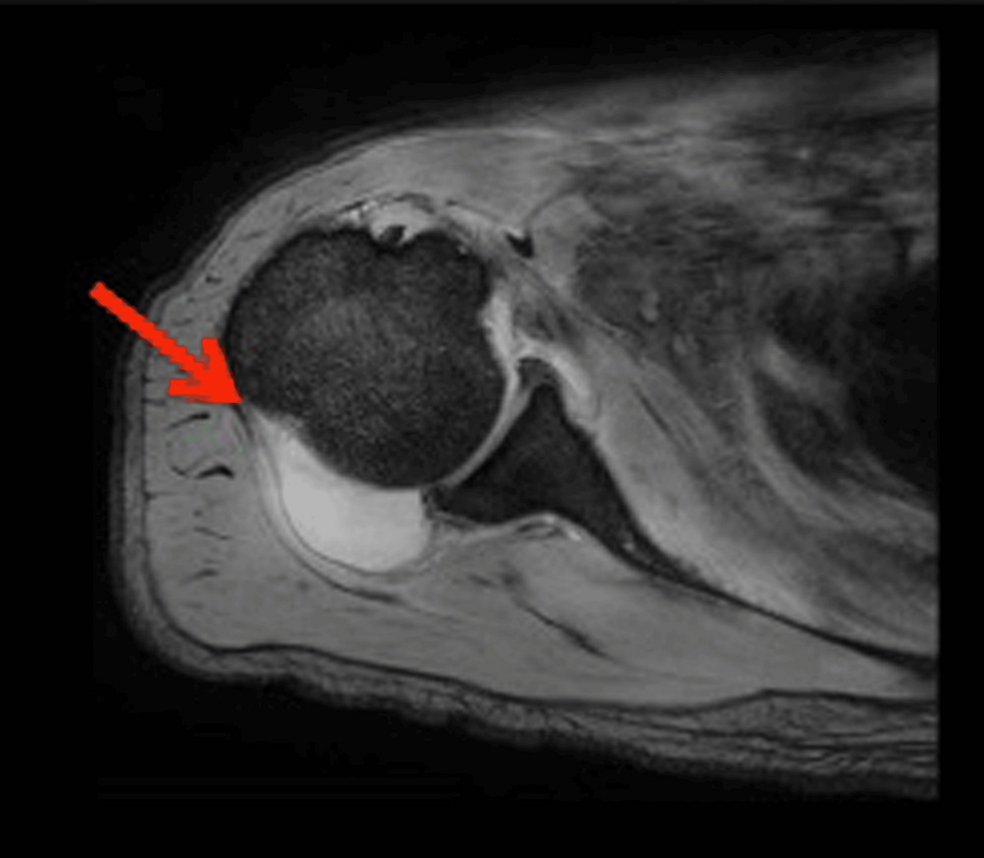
Shoulder T2-weighted magnetic resonance imaging showing arthritis with a high signal.
